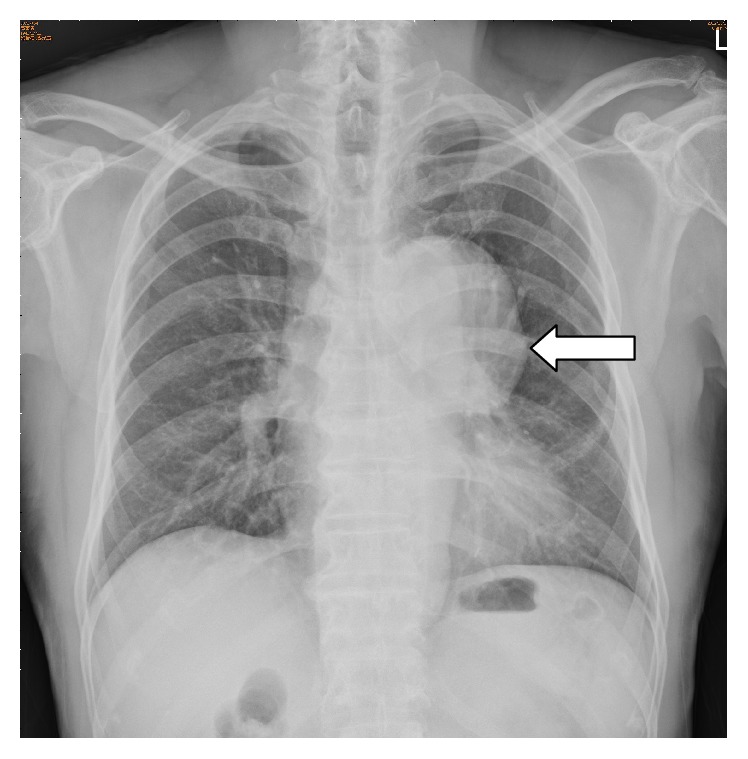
Chest radiography revealed a large bulging contour overlying aorta and left hilar shadow.
